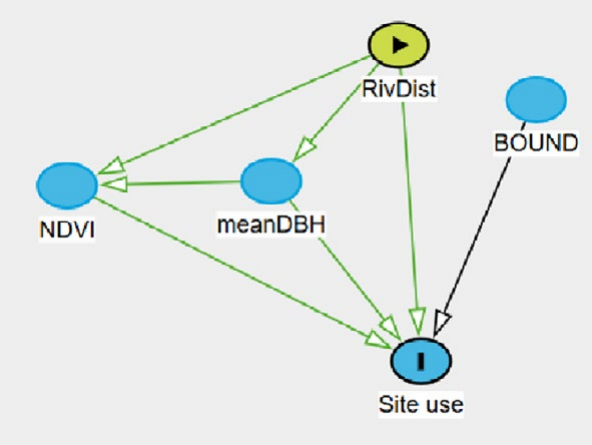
Site use probability	Contact with CT
